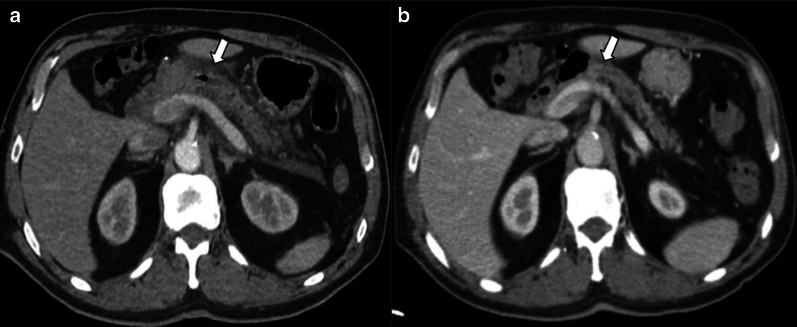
Pancreatojejunostomy stenosis and recurrent pancreatitis (a) 72-year-old male patient with history of pancreaticoduodenectomy due to chronic pancreatitis in the setting of acute stump pancreatitis (big arrow). Endoscopic ultrasound revealed pancreaticojejunostomy with decreased caliber. (b) Control CT scan performed three months later denote normal wirsung
